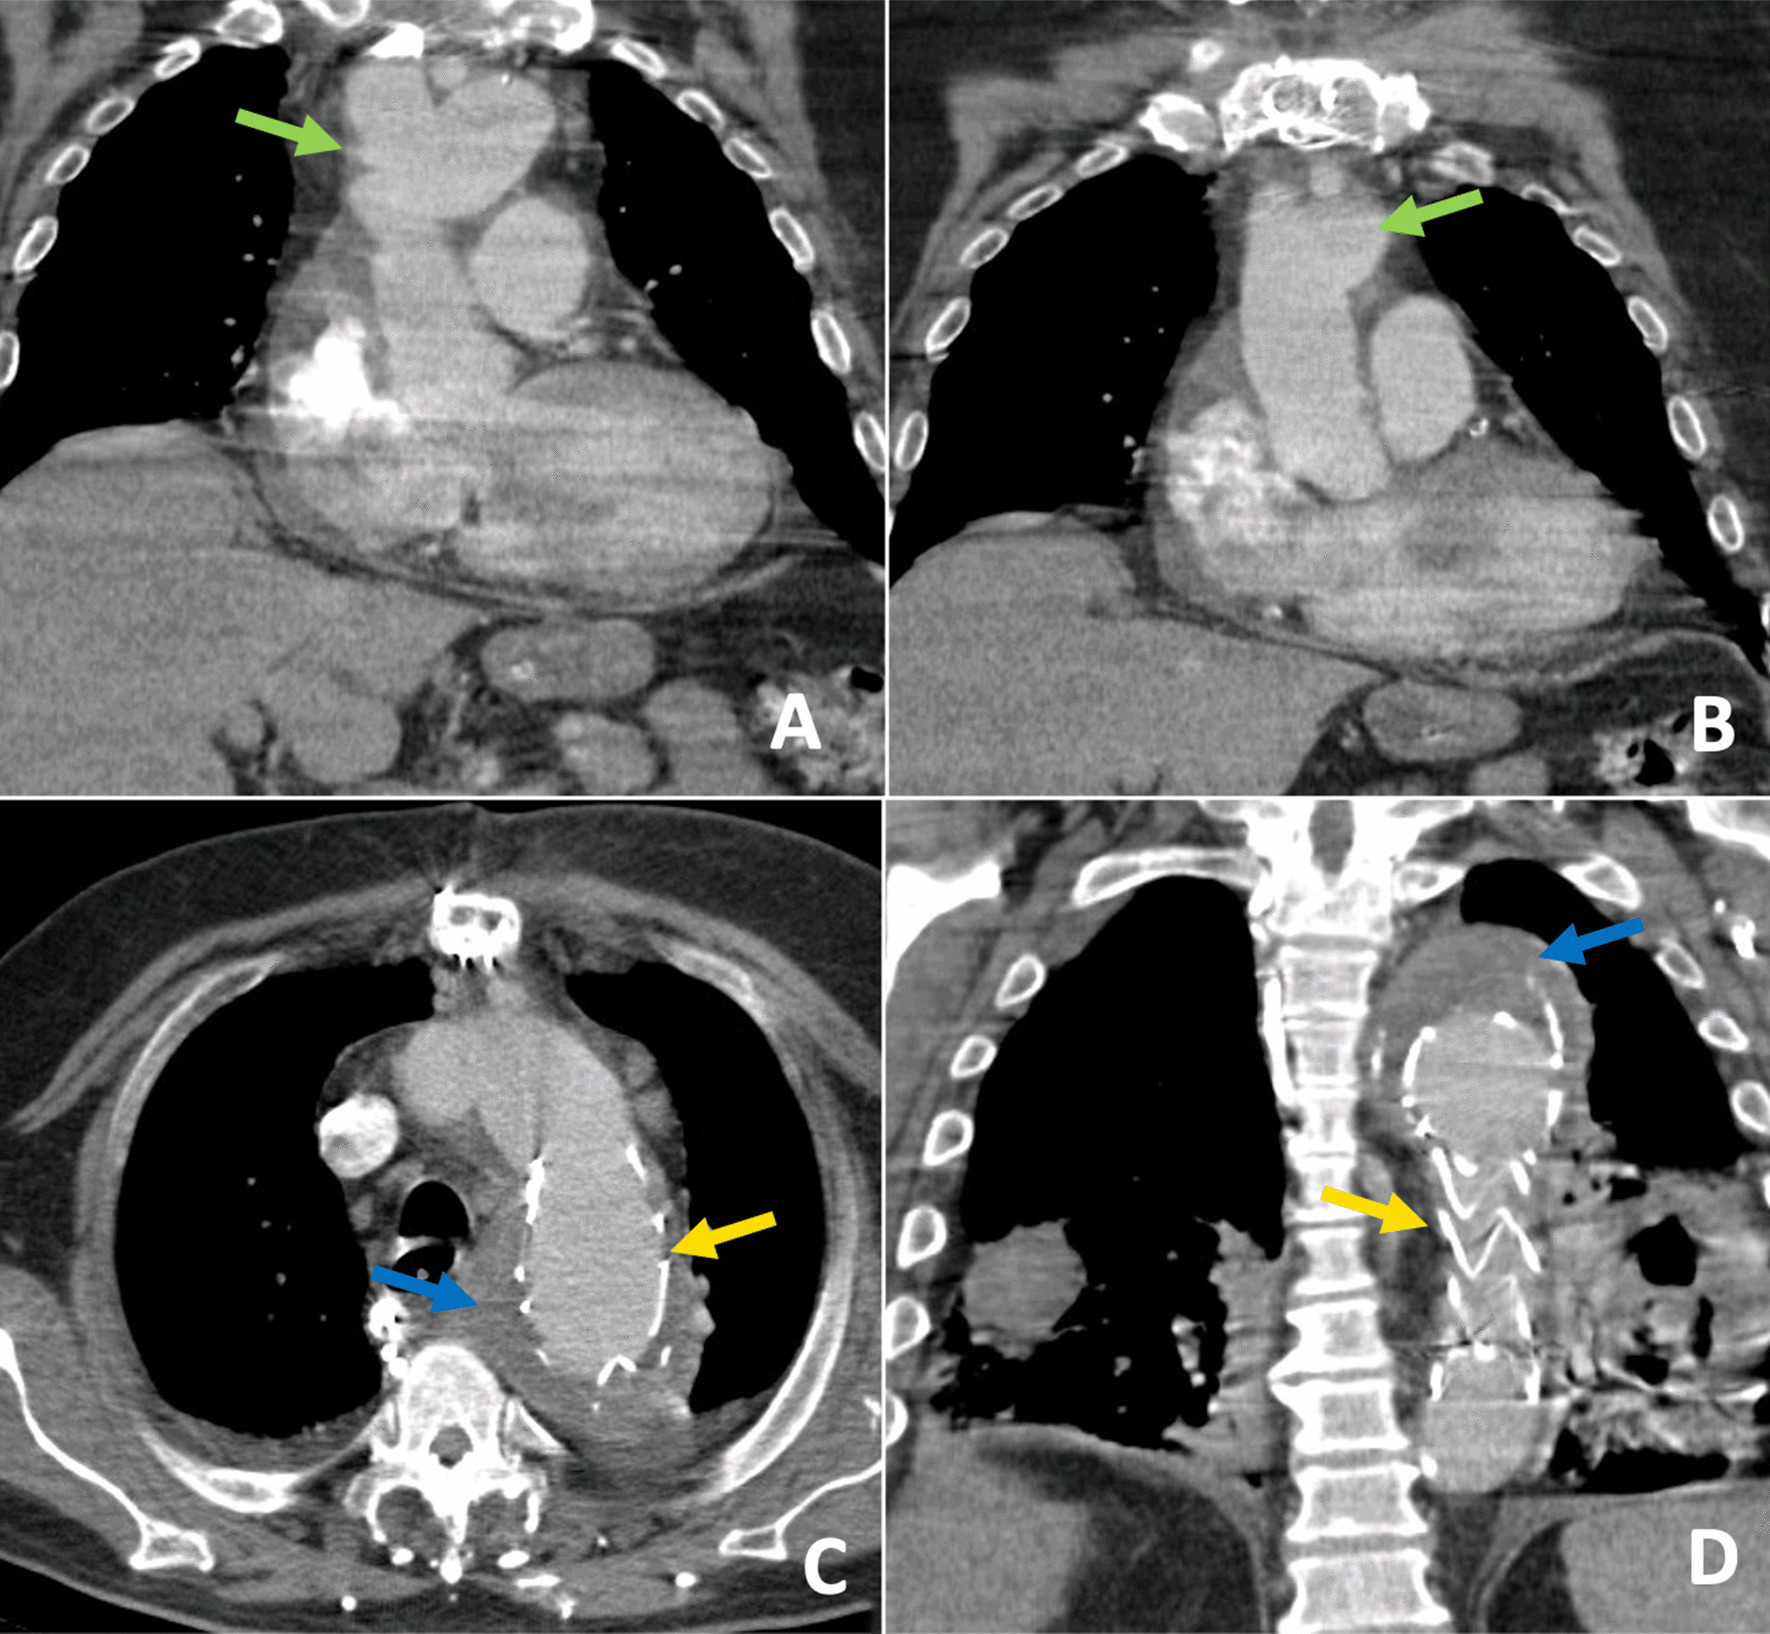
Case 1, non-gated postoperative chest CT at five years follow-up. A, B, and D Coronal images show appropriate positioning and patency of the thoracic graft with no further dilation or other complications (yellow arrows on C and D). A correct re-implantation of the supra-aortic trunks is also visualised (green arrows on A and B). A thrombosed aneurysmal sac is also evidenced (blue arrows on C and D). D Note the predominantly bi-basal opacities in the context of community-acquired pneumonia
